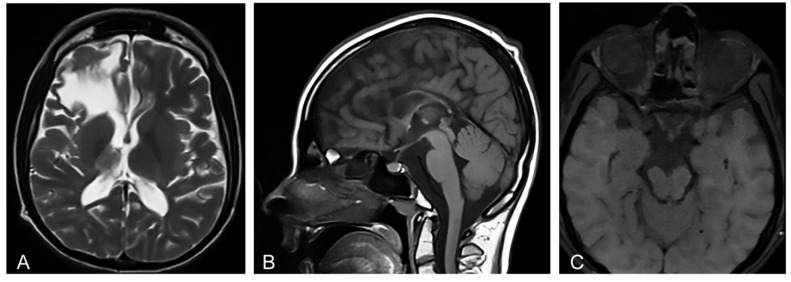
(A) Proband’s axial T2-weighted Magnetic resonance image (MRI) at the age of 20 shows abnormalities in supratentorial white matter and thalamus, and a porencephalic cyst in the right frontal lobe with the compression on the right lateral ventricle. (B) Proband’s sagittal T1-weighted MRI shows microcephaly and hypoplasia of the corpus callosum secondary to white matter loss. (C) Proband’s axial T1-weighted MRI shows an atrophy of the optic nerves, optic tracts, and optic chiasm.
